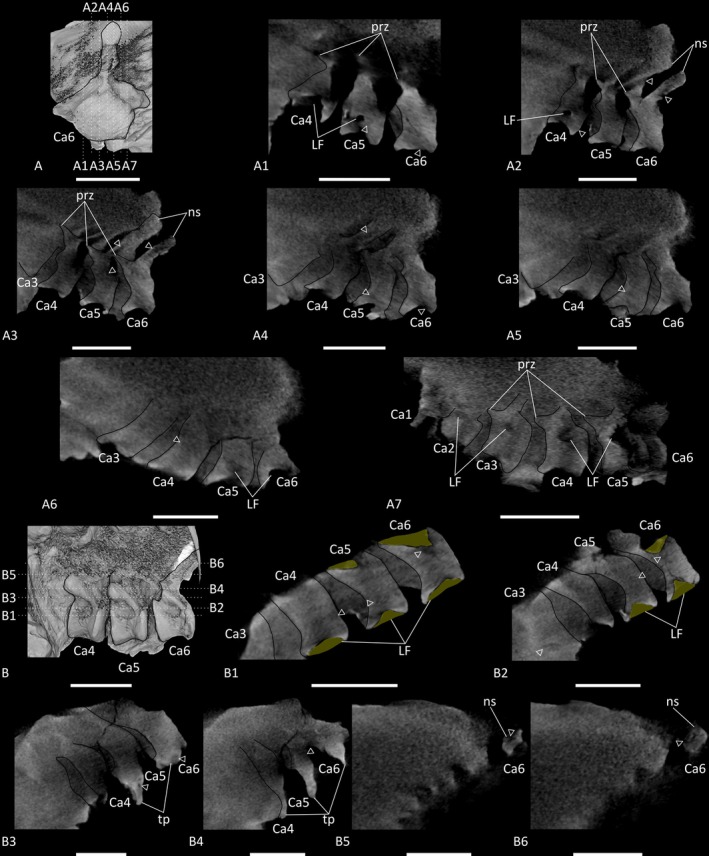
CT scan of *Barosaurus* sp. DINO 2921 anterior caudal vertebrae 1–6. (A, B) 3D rendering (A) posterior view, (B) left lateral view; (A1–A7) CT scan slices in parasagittal cross‐section, (B1–B6) CT scan slices in horizontal cross‐section. Ca, caudal vertebra; LF, lateral fossa; ns, neural spine; prz, prezygapophysis; tp, transverse process. Key: Yellow highlight, centrum fossa; white arrow, pneumatic bone texture. Scale bars = 100 mm.
